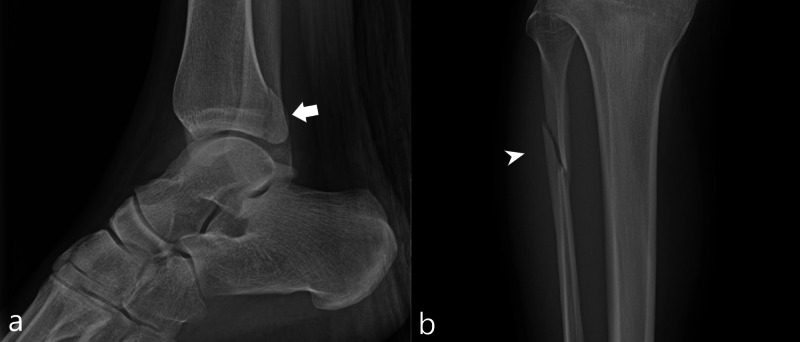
Case 2 ankle and tibia/fibula radiographs Figure 2: A lateral radiograph of the ankle (a) demonstrates a nondisplaced posterior malleolar fracture (white arrow). A frontal radiograph of the tibia (b) demonstrates an oblique fracture of the proximal fibular diaphysis (white arrowhead).
